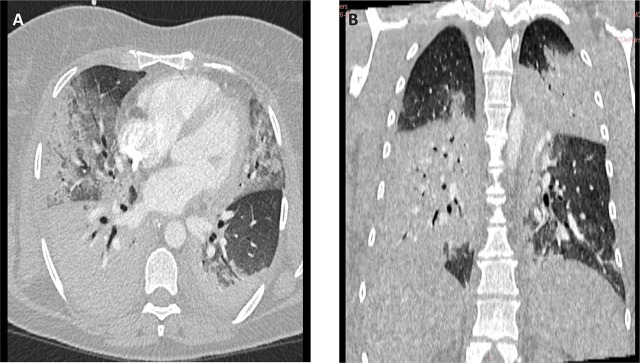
A. Thoracic CT angiography, axial section, acquired with intravenous contrast, pulmonary window. B. Thoracic CT angiography, coronal section, acquired with intravenous contrast, pulmonary window.
